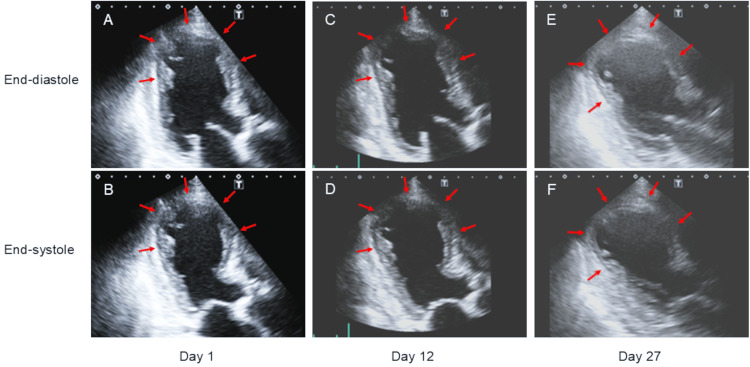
Serial echocardiographic images. Transthoracic echocardiography demonstrates extensive akinesis of the left ventricular (LV) apical segments, extending beyond the territory of a single coronary artery, with an ejection fraction of 35% (A and B, arrows). Follow-up echocardiography was performed weekly, demonstrating no significant recovery of LV wall motion throughout the hospital course (C-F, arrows). The LV ejection fraction remained between 35% and 40% during the hospital course, with no significant improvement.
